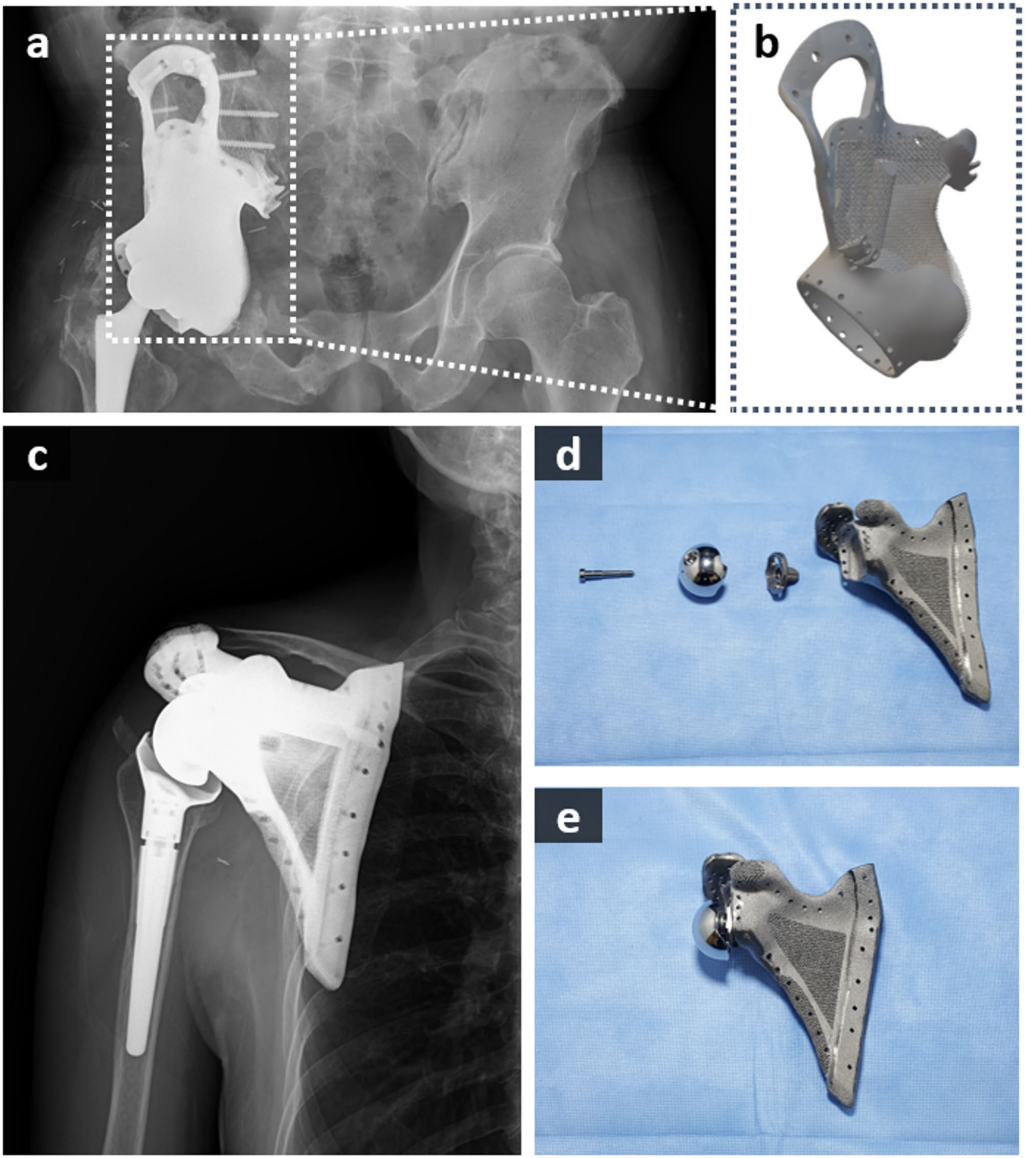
(a, c) X-ray images of patients after implant surgery (b, d, e) Images of the 3D-printed implants (b pelvis, d-e scapula) used by the patients.
